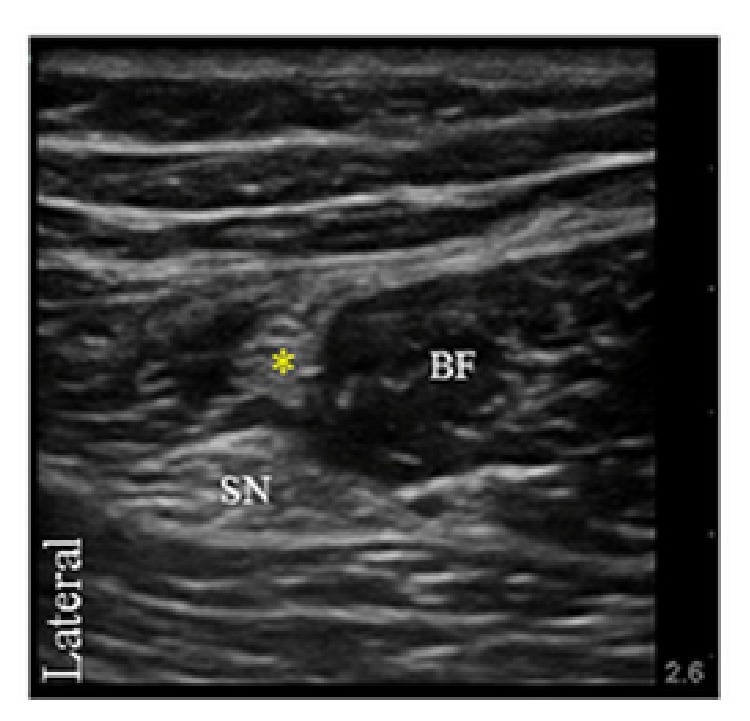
Transverse ultrasound image of the posterior femoral cutaneous nerve (asterisk) located deep to the gluteus maximus muscle and lateral to the biceps femoris muscle (BF). Sciatic nerve (SN).
